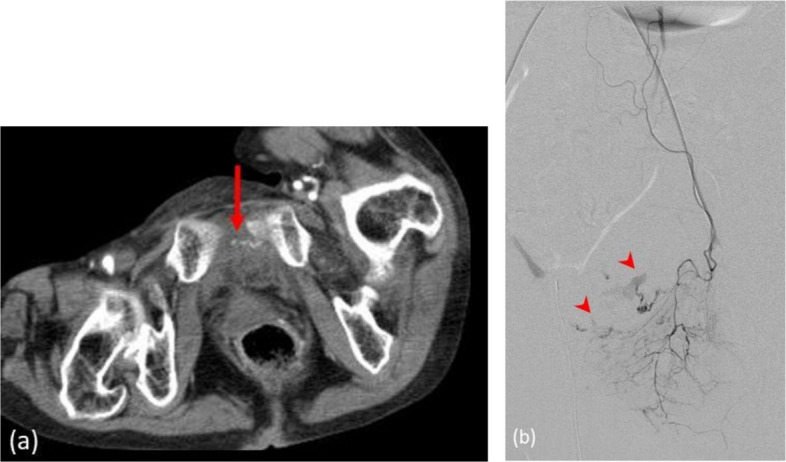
A 72-year-old woman presented with hemodynamic instability with multiple injuries after a traffic accident. a Contrast-enhanced computed tomography demonstrated active contrast extravasation adjacent to a left pubic ramus fracture (arrow). b Angiography revealed extravasation from a pubic branch supplied via an anastomosis between a descending branch of the left inferior epigastric artery and the pubic branch (arrowheads). Transcatheter arterial embolization was performed using gelatin sponge
